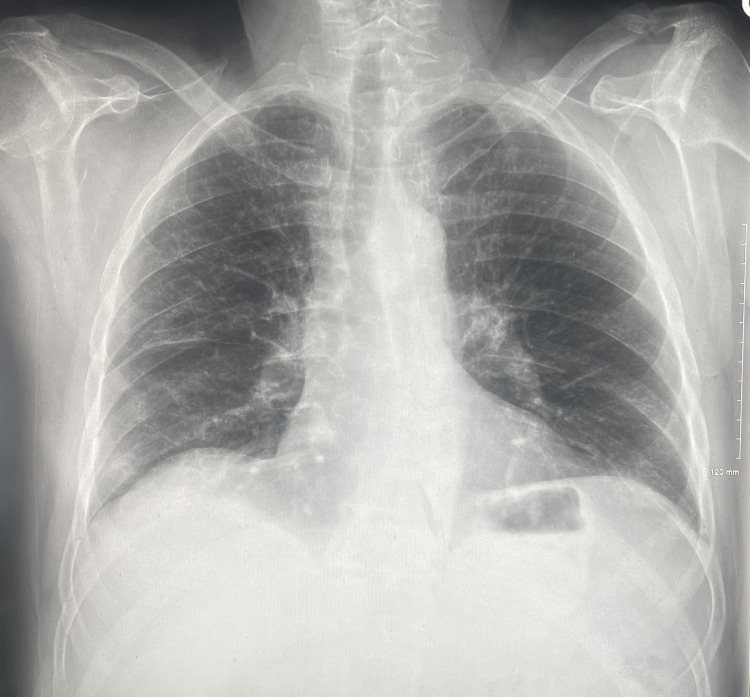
Initial chest X-ray in the ER displaying bilateral, peripheral, patchy airspace opacities.
